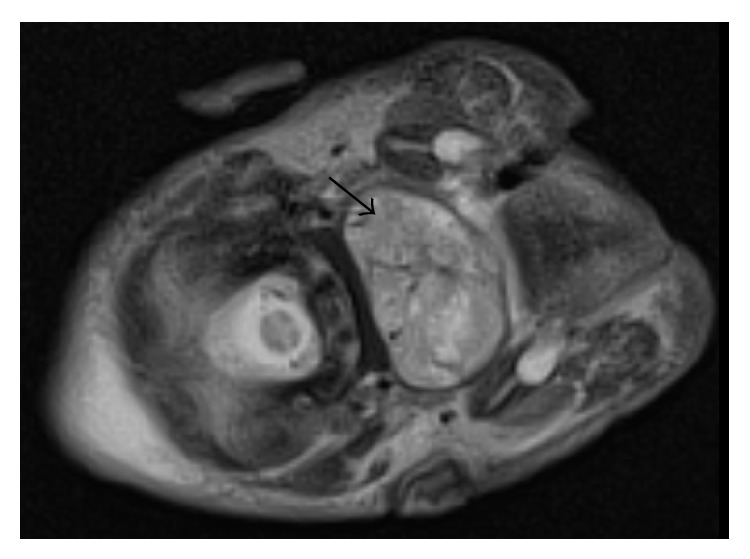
T2 weighted MRI of NPT (axial view).
